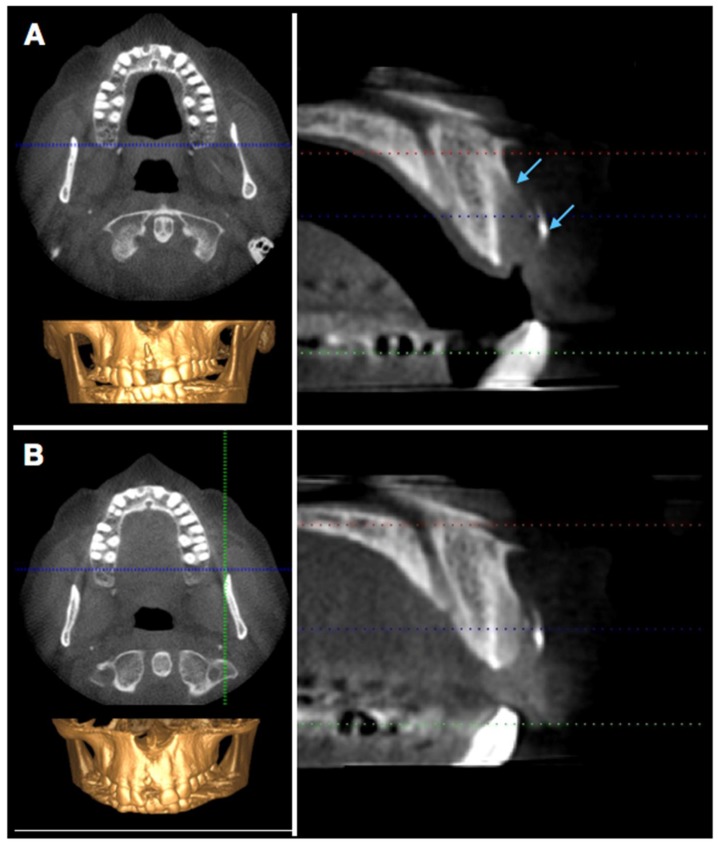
Radiographic evaluation (CBCT) after 6 (A) and 16 (B) weeks. The arrows in panel (A) indicate the border of the buccal bone plate and the radiopaque membrane.
